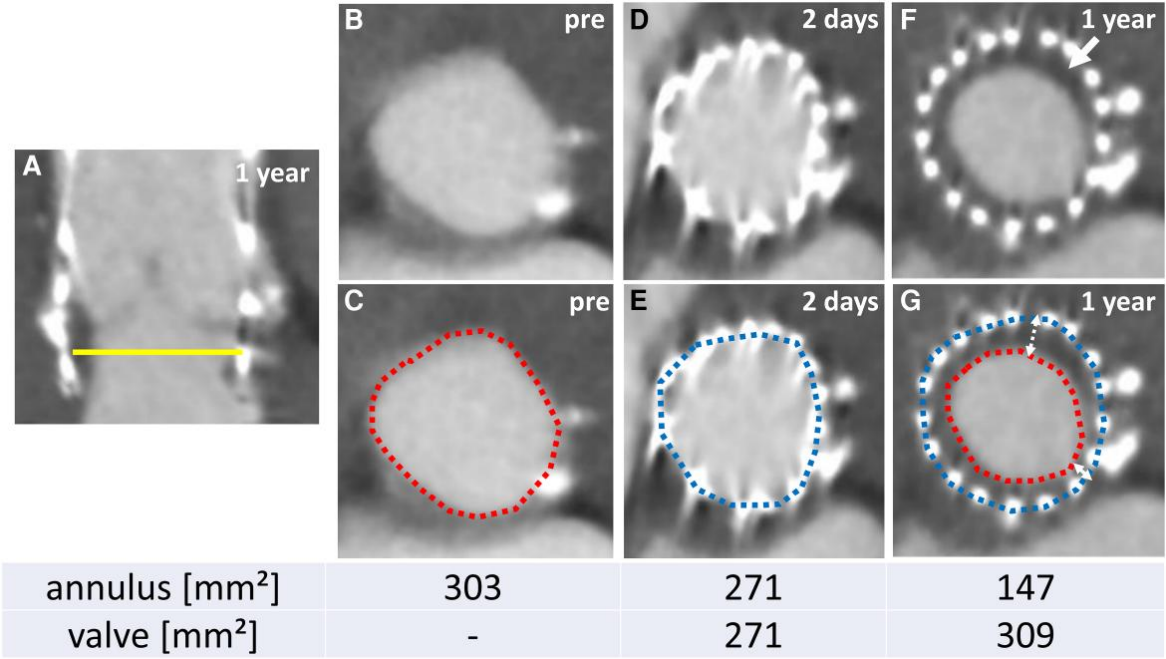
Computed tomography measurements. (*A*) Long-axis 1 year after transcatheter aortic valve implantation. (*B*–*G*) Short axis at the height of the yellow line. (*B*, *C*) Preoperative. (*D*, *E*) Two days after transcatheter aortic valve implantation. (*F*, *G*) One year after transcatheter aortic valve implantation. Red and blue lines indicate annulus and valve sizes, respectively; the white arrow in (*F*) indicates hypo-attenuated annular narrowing; the dotted double arrow in (*G*) indicates the distance between the valve and annulus.
